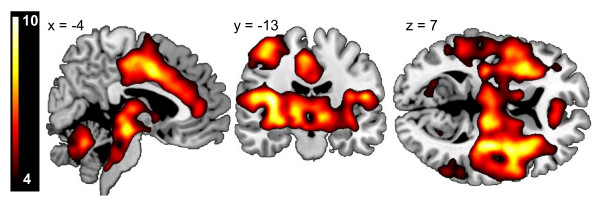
Main effect of unconditioned responses. Unconditioned stimulus (US) versus negative conditioned stimulus (CS)- offset in subjects with post-traumatic stress disorder (PTSD) and trauma-exposed non-PTSD control (TENC) subjects. Although the US was 'highly annoying' rather than painful, it elicited massive neuronal responses in classic pain regions including the brainstem, the thalamus, the contralateral sensory cortices, the insula and the middle cingulate. Activations are displayed at a threshold of T > 4 on a template magnetic resonance imaging (MRI) scan.
